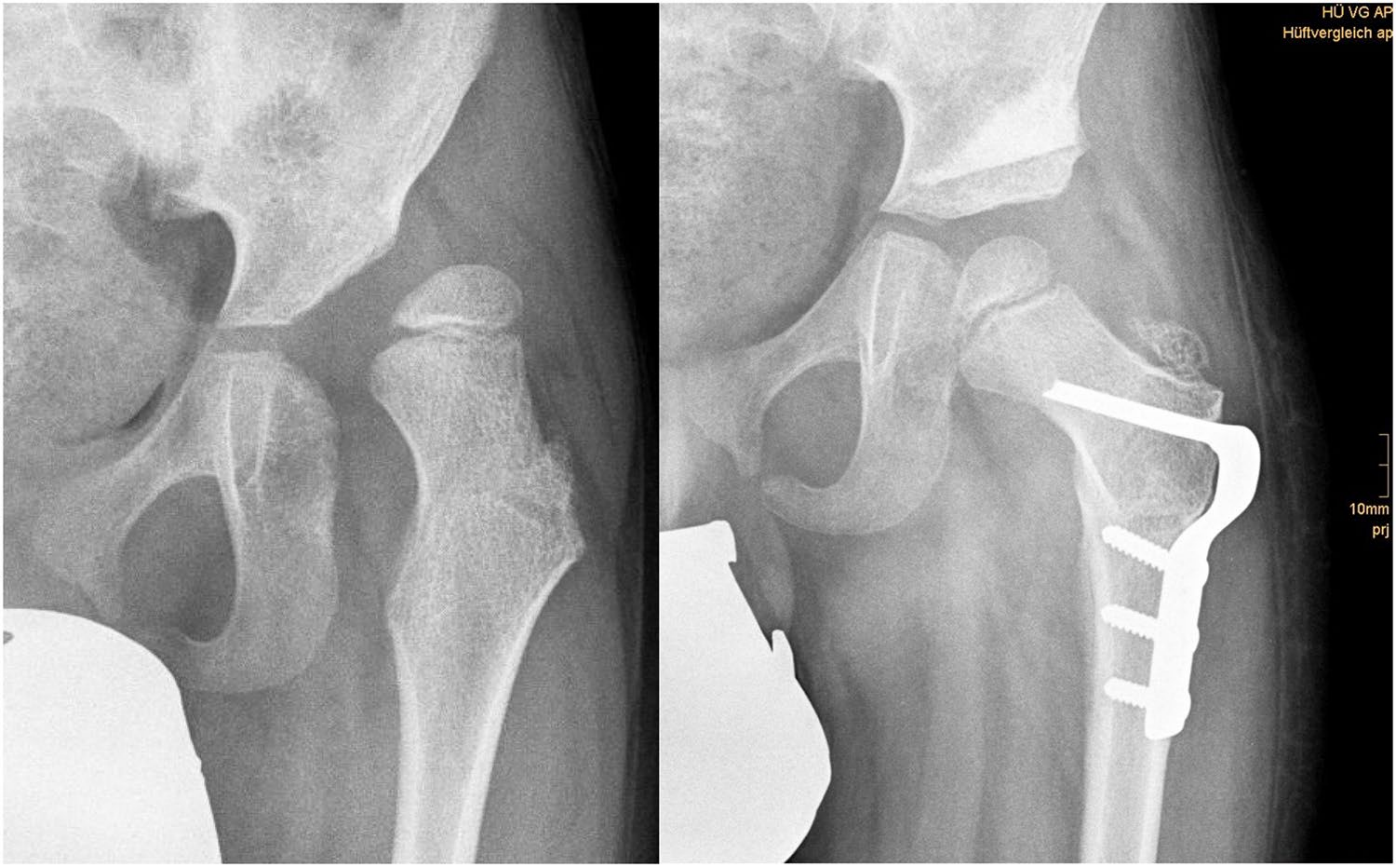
Anteriorposterior X-rays of a 6-year-old boy with neurogenic hip dislocation with excellent results 3 months postoperatively
